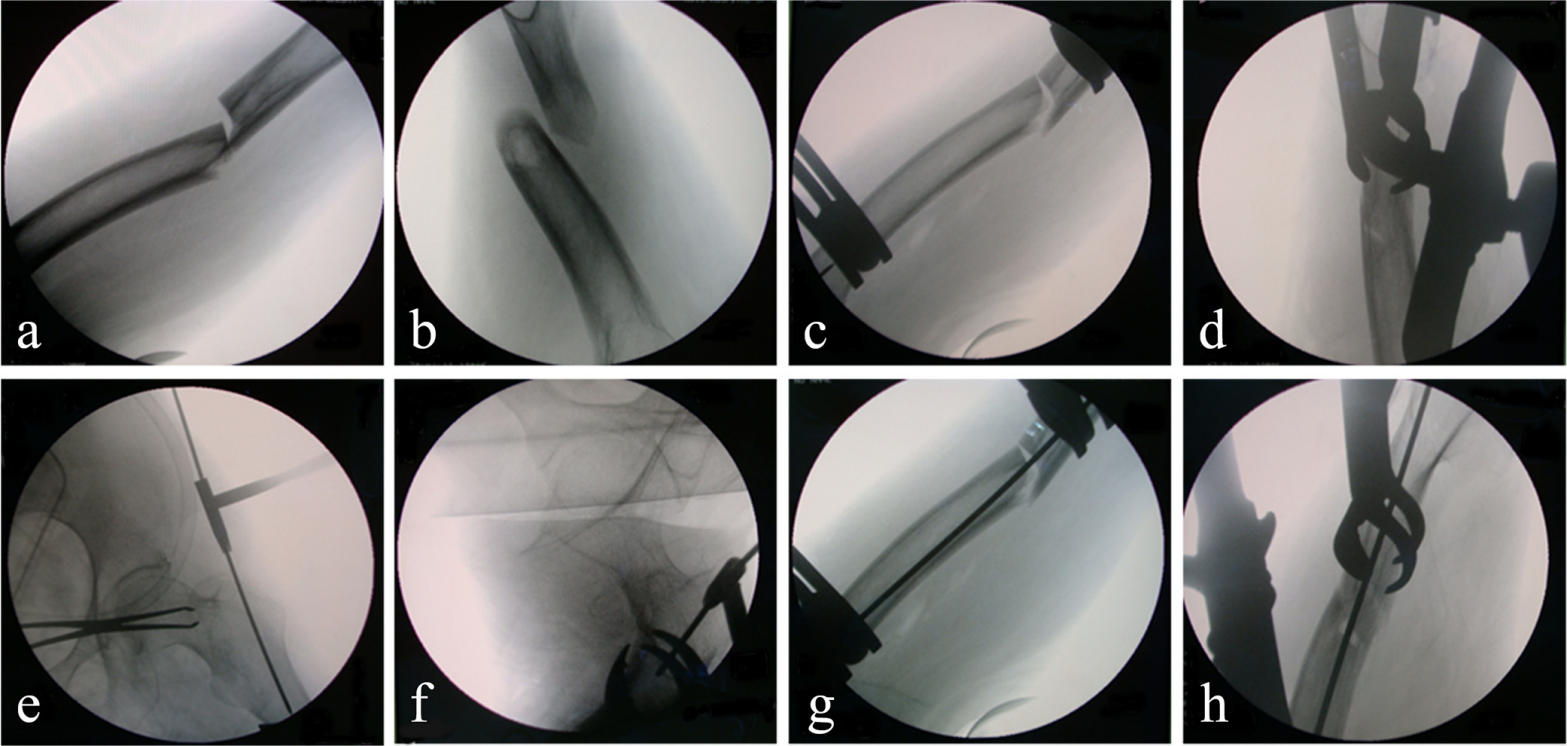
Radiographs showing application of the instrument in an operation for a femoral shaft fracture. (a, b) preoperative condition of the fracture (c, d) completed closed reduction with fracture reduction device (e, f) drilling into the proximal femoral medullary cavity using a Kirschner wire (1.5 mm) through the short sleeve (g, h) drilling into the distal femoral medullary cavity using a Kirschner wire.
